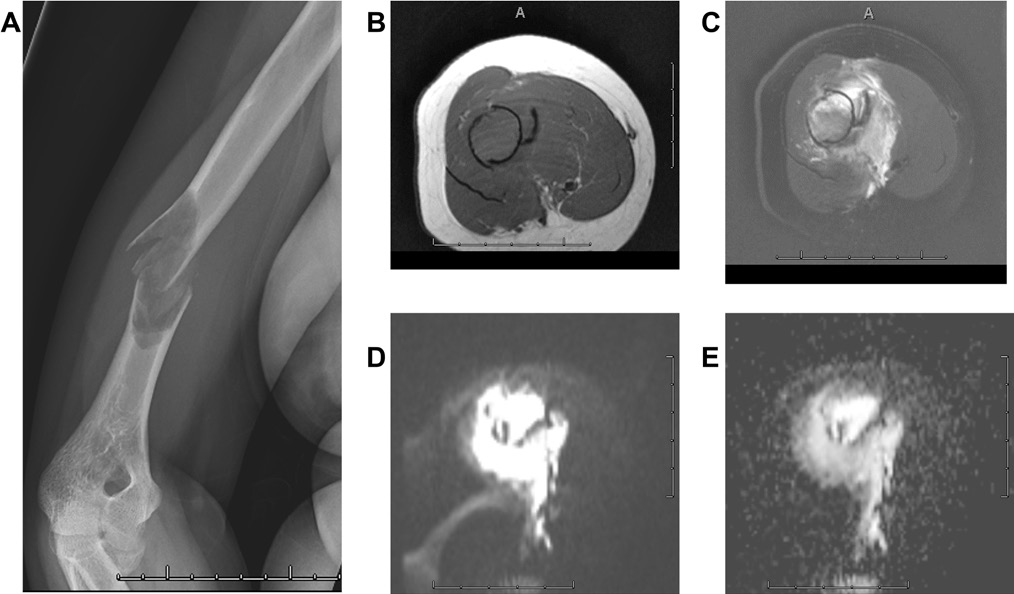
Bone cyst in a middle-aged person (A) X-ray of a humerus shows a pathologic bone fracture with a lucent lesion in the distal shaft. MRI shows an isointense lesion on *T*
